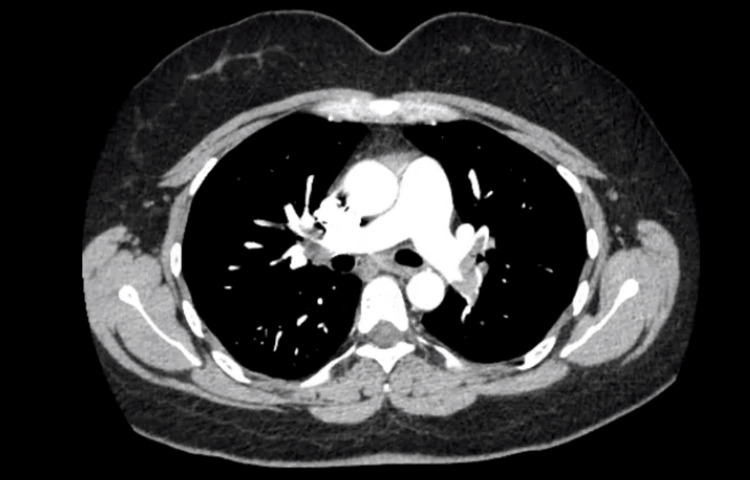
CT angiogram showed large bilateral central pulmonary arteries extending into the lobar and segmental vessels bilaterally with no evidence of right heart strain CT: computed tomography
